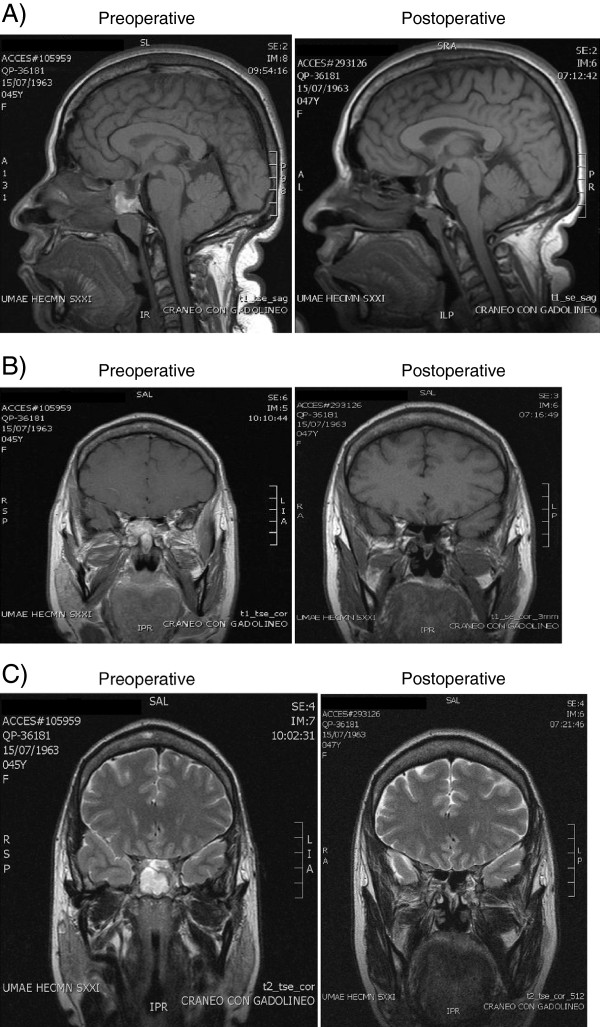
Preoperative (left) and postoperative (right) MRI. A) T1W sagital, gadolinium-enhanced; B) T1W coronal, gadolinium-enhanced; C) T2W coronal.
